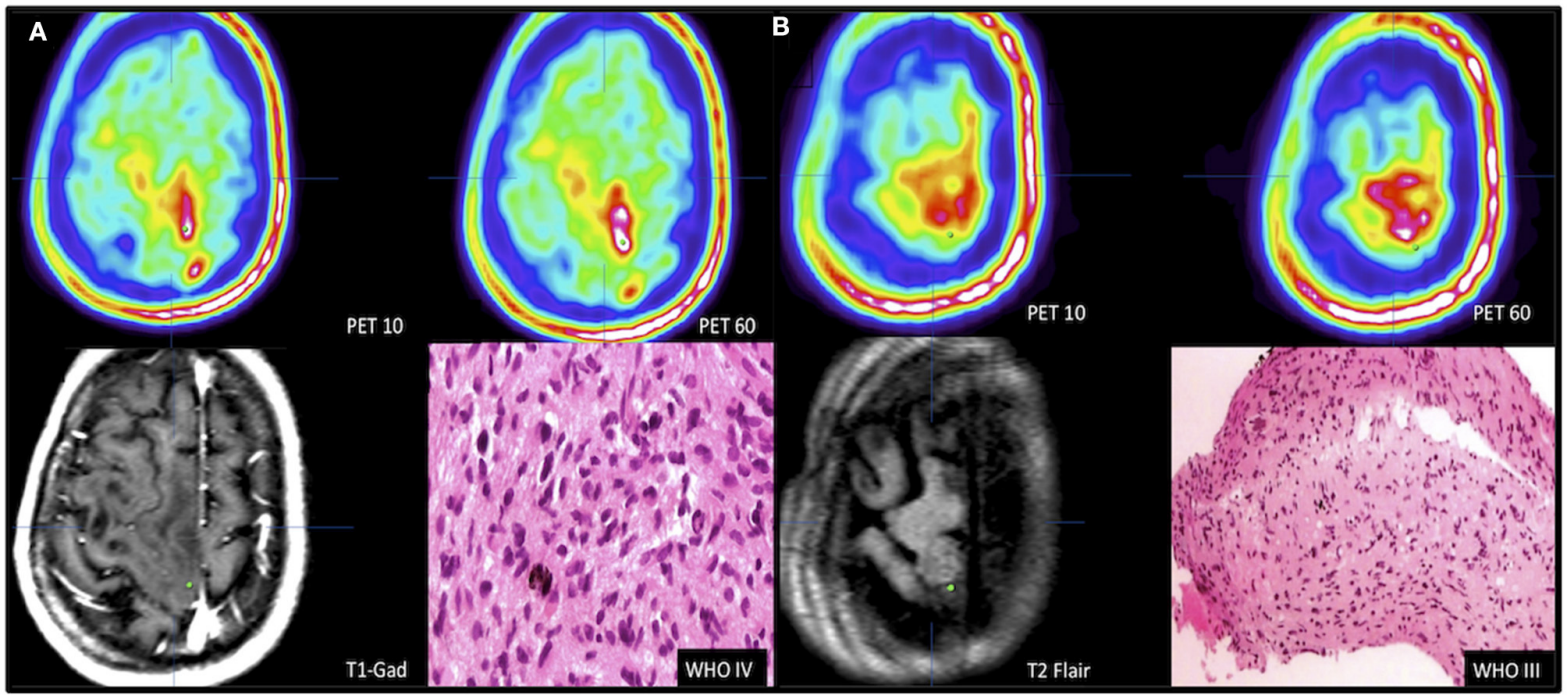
Selection of biopsy target based on dual time point. Patient 2 presented with tumor of eloquent area. Target of highest malignancy (A) was selected using FET kinetics defined in dual time-point PET images with no contrast enhancement in the T1-CE sequence. PET10 lesion defined by FET uptake hotspot (1.88 SUV) was expected to be highly malignant (white area); PET60 lesion defined by coexistence of hotspots (white areas) (2.64 SUV). Biopsy target marked by a green dot. Biopsy from hotspot of early occurrence showed GBM. (B) Defining the tumor border by targeting the tumor periphery (green dot, PET10 1.26 SUV and PET60 1.1 SUV) in area of moderate FET uptake visible on dual time-point PET with no contrast enhancement in T1+gadolinum sequence and no clear changes visible on T2-FLAIR MRI image. Biopsy result was grade III astrocytoma.
